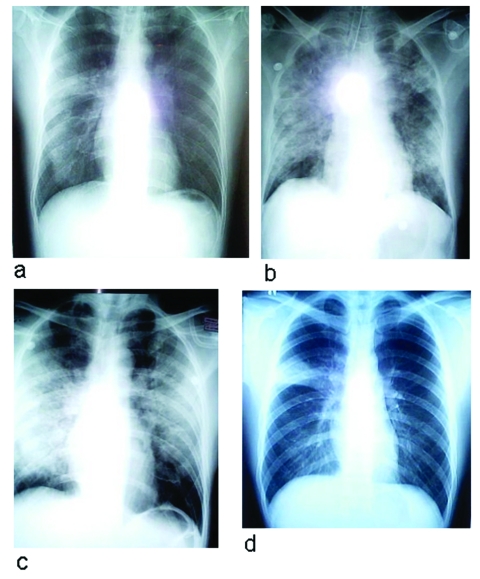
Chest radiographs of two patients with severe acute respiratory syndrome (SARS). a–c: radiographs of patient 5 showing progression of changes. a, day 8 of symptoms; b, day 13 of symptoms, d, day 14 of symptoms. He died on day 19 of this illness. d, chest radiograph, taken on day 8 of symptoms, of patient 12, with right upper lobe infiltrates resembling pulmonary tuberculosis (TB) but laryngeal swab cultures for TB were negative.
